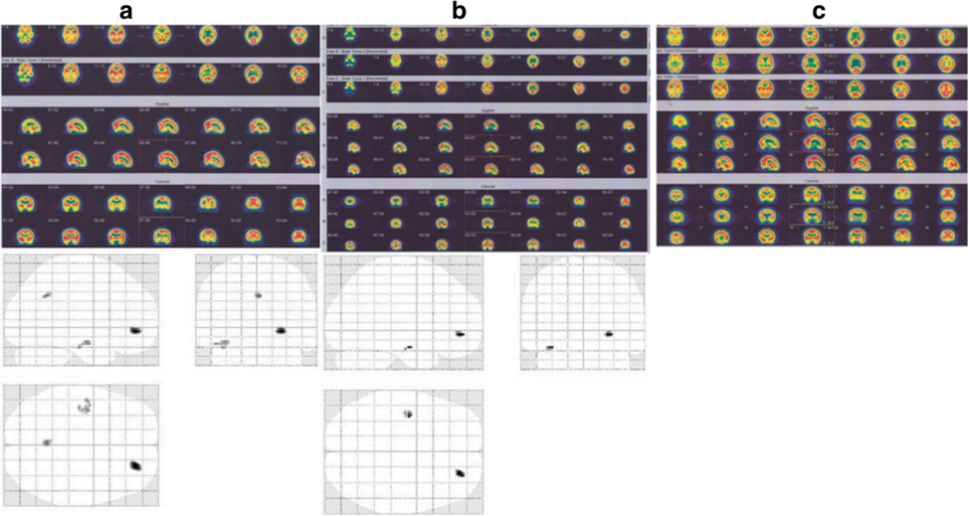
Brain Perfusion SPECT and statistical parametric mapping analysis of a MS patient underwent HBOT. First brain perfusion study of MS woman patient showed moderate hypoperfusion of inferior temporal lobe on the right side (a). Second brain perfusion study 33 days after first study and following first oxygen therapy showed mild and moderate hypoperfusion of inferior temporal lobe on the right side at first scan (no significant change in visual analysis) (b). Third brain perfusion study 4.4 months after second study and following second oxygen therapy showed rather normal radiotracer distribution through the cortical and subcortical regions (significant changes on visual analysis) (c). The upper rows indicate transverse 99mTc-ECD SPECT images; the middle rows indicate sagittal 99mTc-ECD SPECT images; and the lower rows indicate coronal 99mTc-ECD SPECT images
